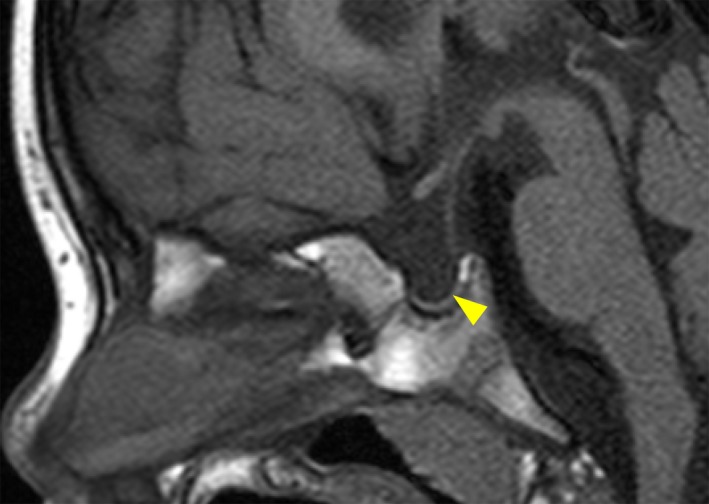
Brain magnetic resonance imaging of the patient at the age of 4 years. A hypoplastic anterior pituitary gland (arrowhead), with a visible but thin stalk, and an eutopic posterior pituitary gland are shown
